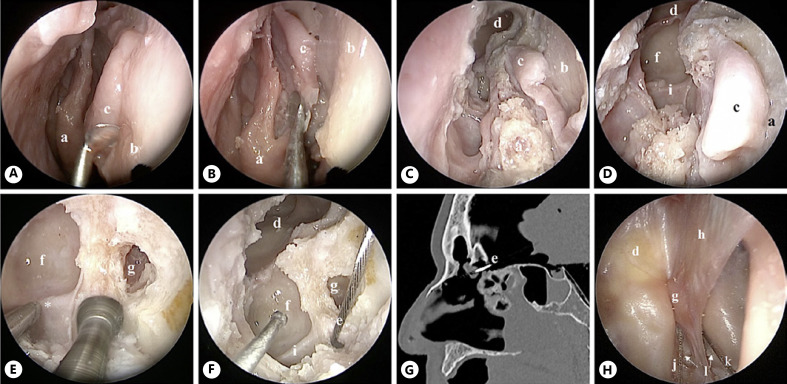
Endoscopic intracranial approach through Draf IIb procedure (approach 4), right nasal fossa. A Septal flap is harvested and positioned safely medially to the middle turbinate (B). C Draf IIb is performed and first fovea ethmoidalis with AEA are exposed (D). E Drilling of the cribriform plate, medially to the cranial insertion of the common basal lamella and posteriorly to the AEA, until the dura is exposed. F Positioning of the electrode; which will be covered with the septal flap afterward. G Sagittal CT scan showing the intracranial positioning of the electrode. H View from above (a craniotomy was performed with endoscopic assistance) showing the transillumination on the posterior wall of the left frontal sinus and the intracranial placement of the electrodes of approaches 3 and 4. a, middle turbinate; b, nasal septum; c, septal flap; d, frontal sinus; e, electrode; f, first fovea ethmoidalis; g, crista galli; h, falx cerebri; i, anterior ethmoidal artery; j, the electrode of approach 3; k, electrode of approach 4; l, cribriform plate.
